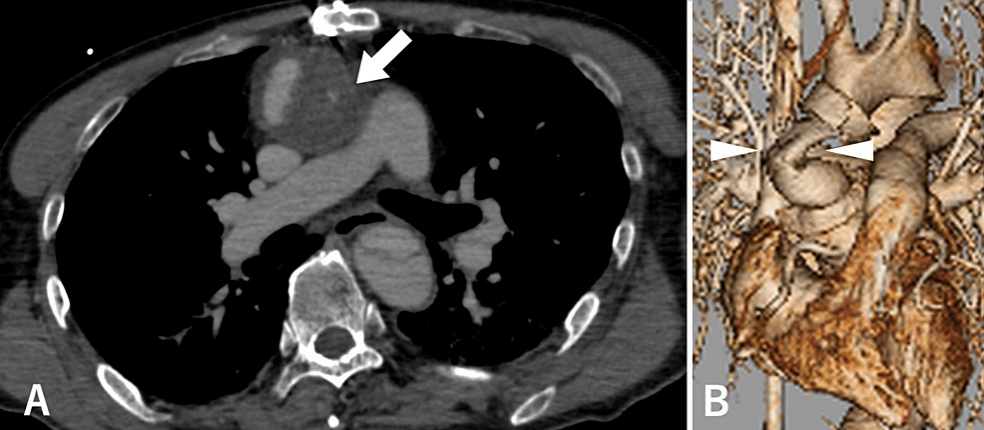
Contrast-enhanced CT scan performed under ECMO. Contrast-enhanced CT scan performed under ECMO support showed extravasation of contrast around the proximal anastomosis site (arrow; A) and the graft kinking induced by hematoma inside the pseudoaneurysm (arrowhead; B). ECMO = extracorporeal membrane oxygenation
